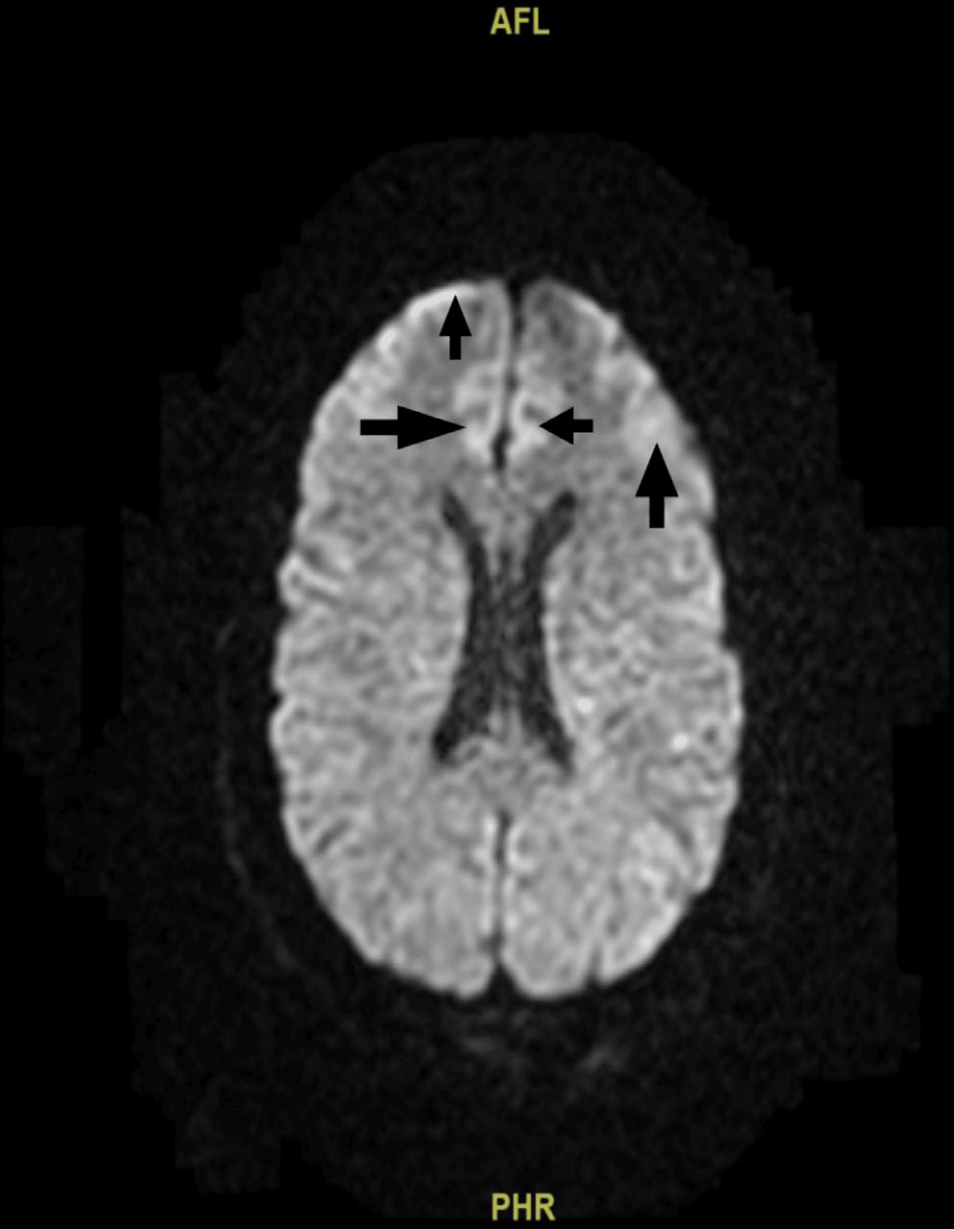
Brain MRI showing bilateral basal ganglia mineralization, mainly in the pallidum, with sparing of the caudate head, and small mineralizations in the frontal lobe white matter, centrum semiovale, and cerebellar hemispheres.
